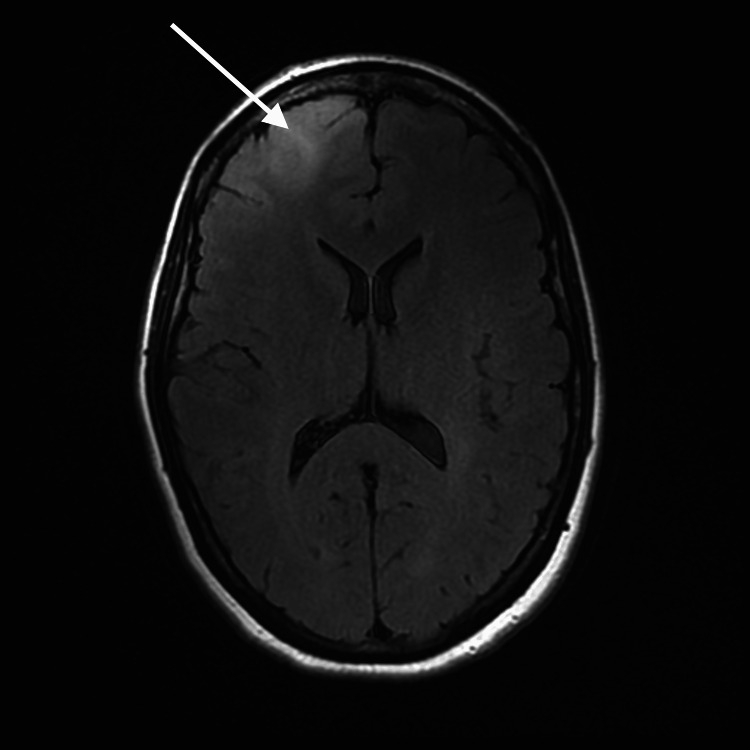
Axial T1 FLAIR demonstrating signal abnormality in the right frontal lobe (arrow) FLAIR: fluid-attenuated inversion recovery
